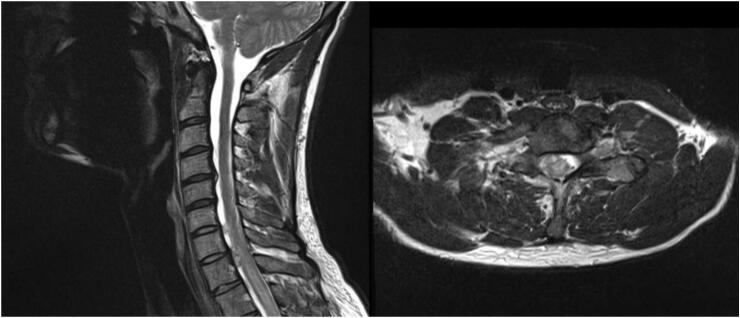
Axial/sagittal T2 weighted MRI revealing ventral compression at the C7-T1 disc space, along with retrovertebral compression.
